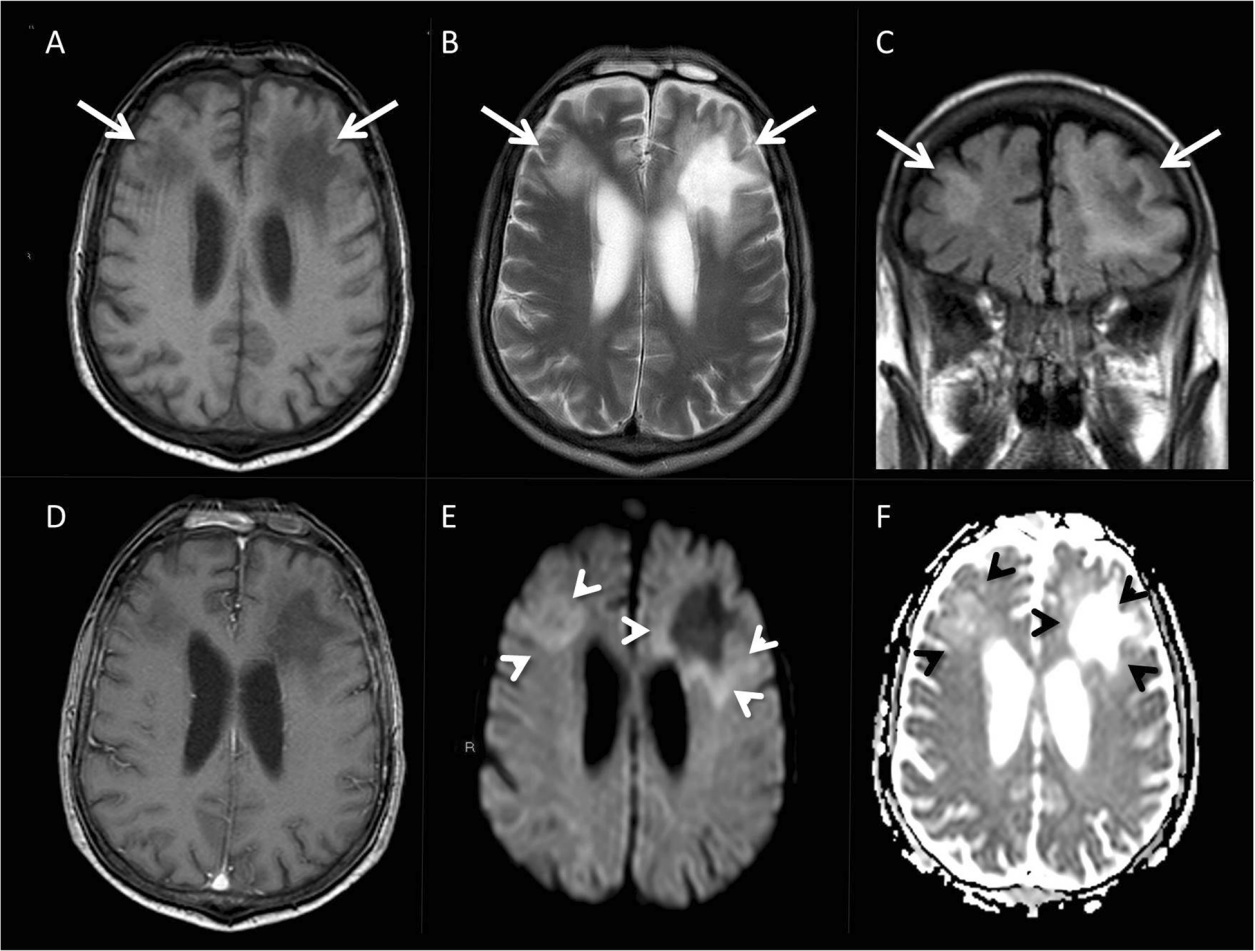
(a) Axial T1-weighted magnetic resonance imaging (MRI) showing asymmetric hypointense areas in the bilateral frontal white matter (arrows). (b, c) They appear hyperintense on the corresponding T2-weighted and FLAIR sequences. (d) The lesions do not enhance following intravenous contrast administration. (e, f) A thin rim of diffusion restriction can be seen along the advancing edge of the lesion on the DWI images and the corresponding ADC maps (arrowheads)
